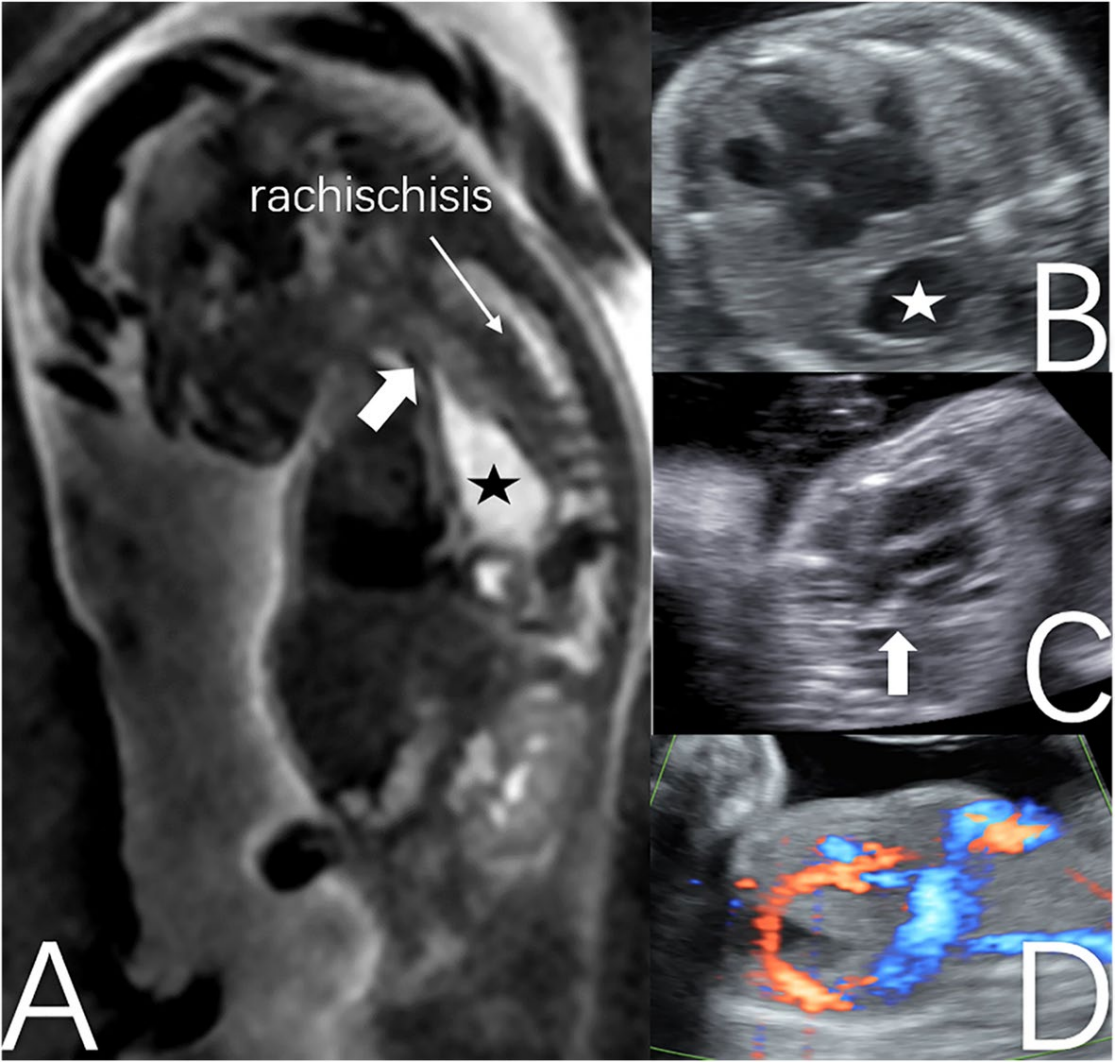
The MR imaging of short oesophagus, intrathoracic stomach, and rachischisis (A). The intrathoracic stomach behind the heart (B). The oesophagus located at the upper edge of the heart (C). Hepatic portal vein passed behind intrathoracic stomach and entered the right atrial (D). (Asteroids refer to stomach, thick arrows refer to oesophagus)
